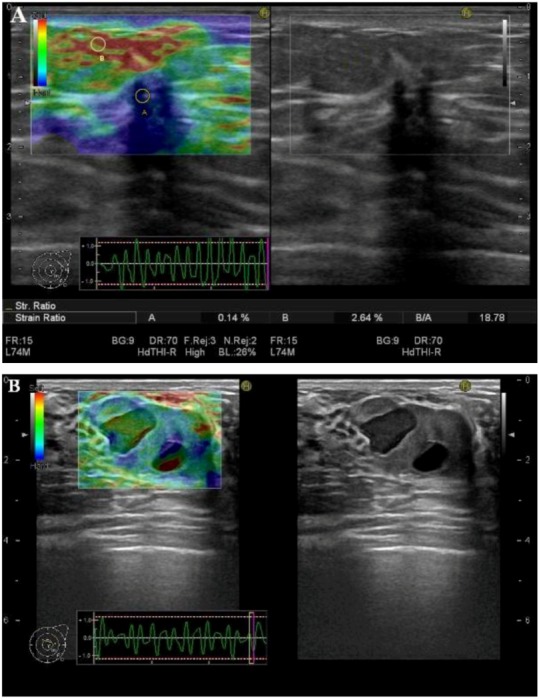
Co-registered Strain elastography (SE) (left) and ultrasound B-mode (right) images of (A) a hard (blue) malignant breast tumor (ductal carcinoma) and (B) a soft (green) benign breast neoplasm (fibroadenoma). The regions of interest (ROIs) placed in the tumor (A) and in the adjacent soft tissues (B) are used to calculate a strain-ratio (SR) (B/A) (right lower corner). The benign neoplasm (B) contains two hypoechoic areas, one of which yields a classic elastogram for a cyst with three color layers (blue-green-red) from top to bottom.
